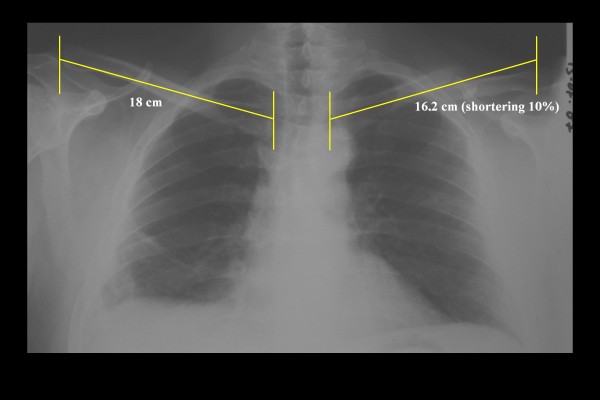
Radiographic image of the clavicle of a dissatisfied patient after conservative treatment of a diaphyseal clavicle fracture. The observed shortening was 18 mm or 10%; the Constant score was 65.
